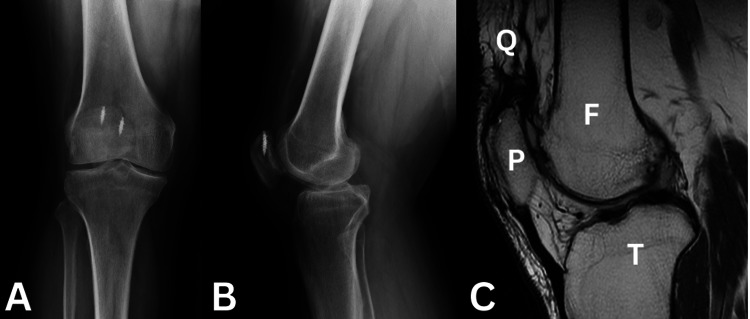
(A) Anteroposterior and (B) lateral X-ray radiographs showing suture anchors in the superior patella pole. (C) A T1 sagittal MRI of the knee with Q showing the quadriceps tear, P showing the patella, F showing the femur, and T showing the tibia.
